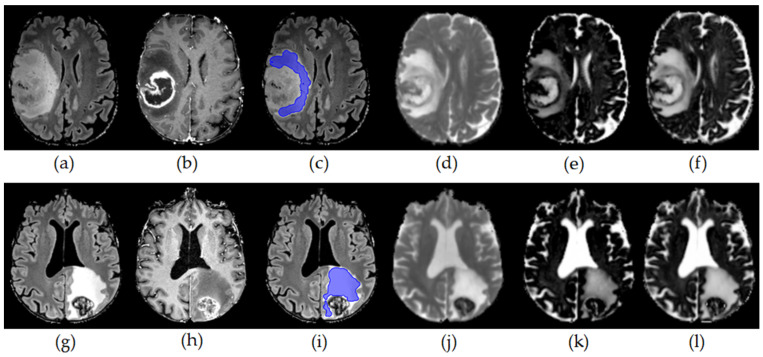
Upper row: Axial FLAIR ((a,c) with superimposed perilesional T2 area segmentation), axial T1 MPRage post-Gd (b) and parametric maps for MD (d), V-ISO (e) and V-CSF (f) in a 60-year-old female patient with a right frontoparietal glioblastoma. Lower row: Axial FLAIR ((g,i) with superimposed perilesional T2 area segmentation), axial T1 MPRage post-Gd (h) and parametric maps for MD (j), V-ISO (k) and V-CSF (l) in a 51-year-old male patient with a left parietal esophageal cancer metastasis.
